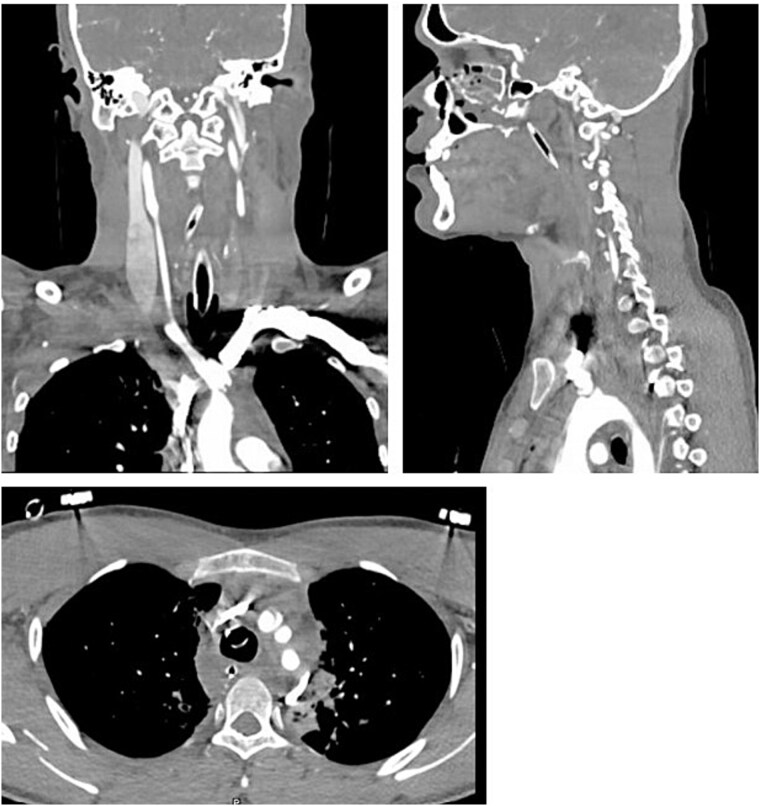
Initial CT scan showing IA flap with tiny extravasation of the contrast.
